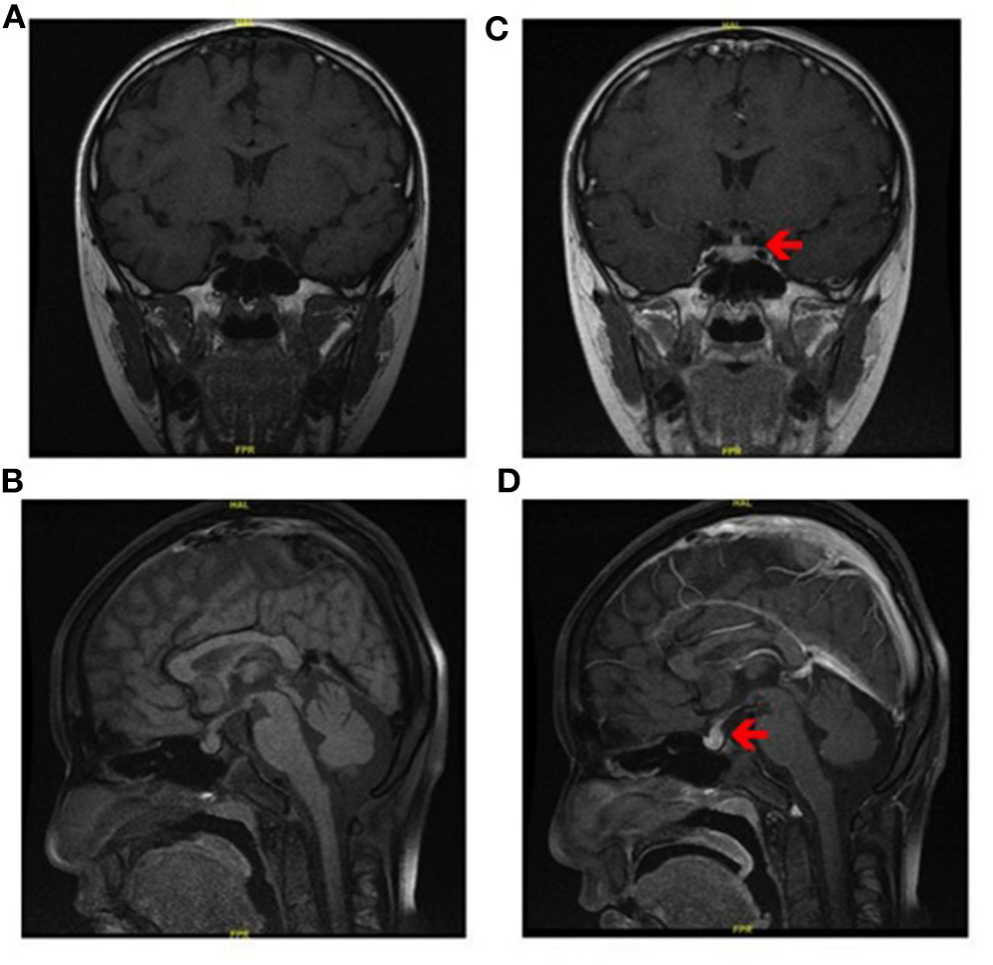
MRI characteristics of Rosai-Dorfman disease involving sellar region. (A) Coronal and (B) sagittal T1-weighted magnetic resonance images. (C) coronal and (D) sagittal contrast-enhanced magnetic resonance images reveal thickened pituitary stalk with obvious homogenous enhancement (red arrow).
